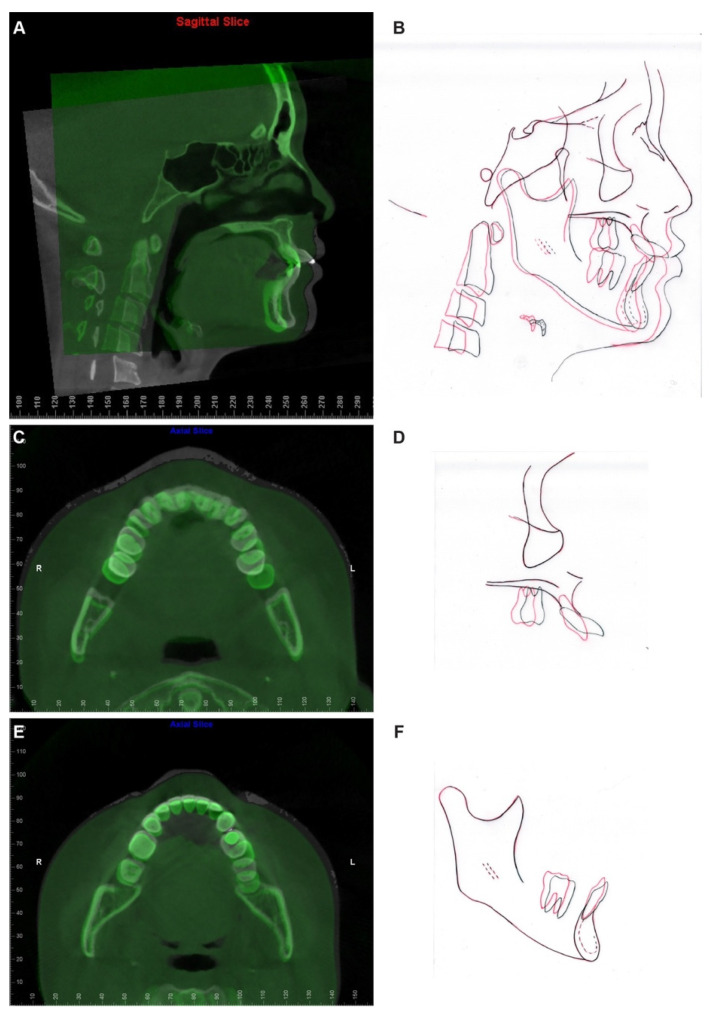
CBCT and cephalometric superimposition. (A) The sagittal slice at the maxillary left central incisor level of pre- (white) and post- (green) treatment CBCT images superimposed based on cranial base. (B) The superimposition of pre- (black) and post- (red) treatment cephalometric tracings superimposed based on cranial base. (C) The Axial slice of pre- (white) and post- (green) treatment CBCT images superimposed based on the cranial base. The slice was captured at the level of the cervical region of maxillary anterior teeth to demonstrate the amount of maxillary molar distalization achieved during treatment. (D) The superimposition of pre- (black) and post- (red) treatment cephalometric tracings of the maxilla. (E) The Axial slice of pre- (white) and post- (green) treatment CBCT images superimposed based on mandibular symphysis. The slice was captured at the level of the cervical region of mandibular anterior teeth to demonstrate the amount of mandibular molar distalization achieved during treatment. (F) The superimposition of pre- (black) and post- (red) treatment cephalometric tracings of the mandible.
